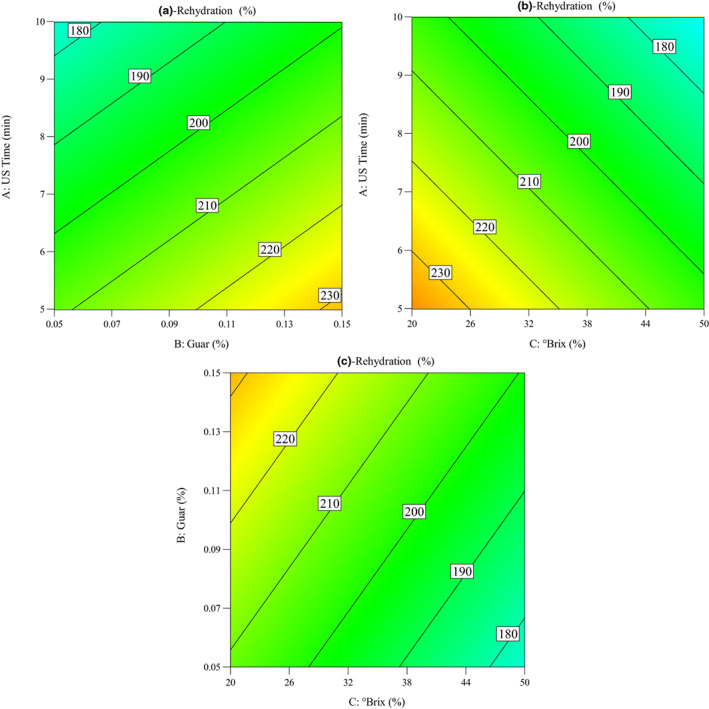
Contour plots for the impacts of operating conditions on the rehydration ratio of quince slices: (a) ultrasound time versus guar concentration, (b) ultrasound time versus °Brix, and (c) guar concentration versus °Brix.
